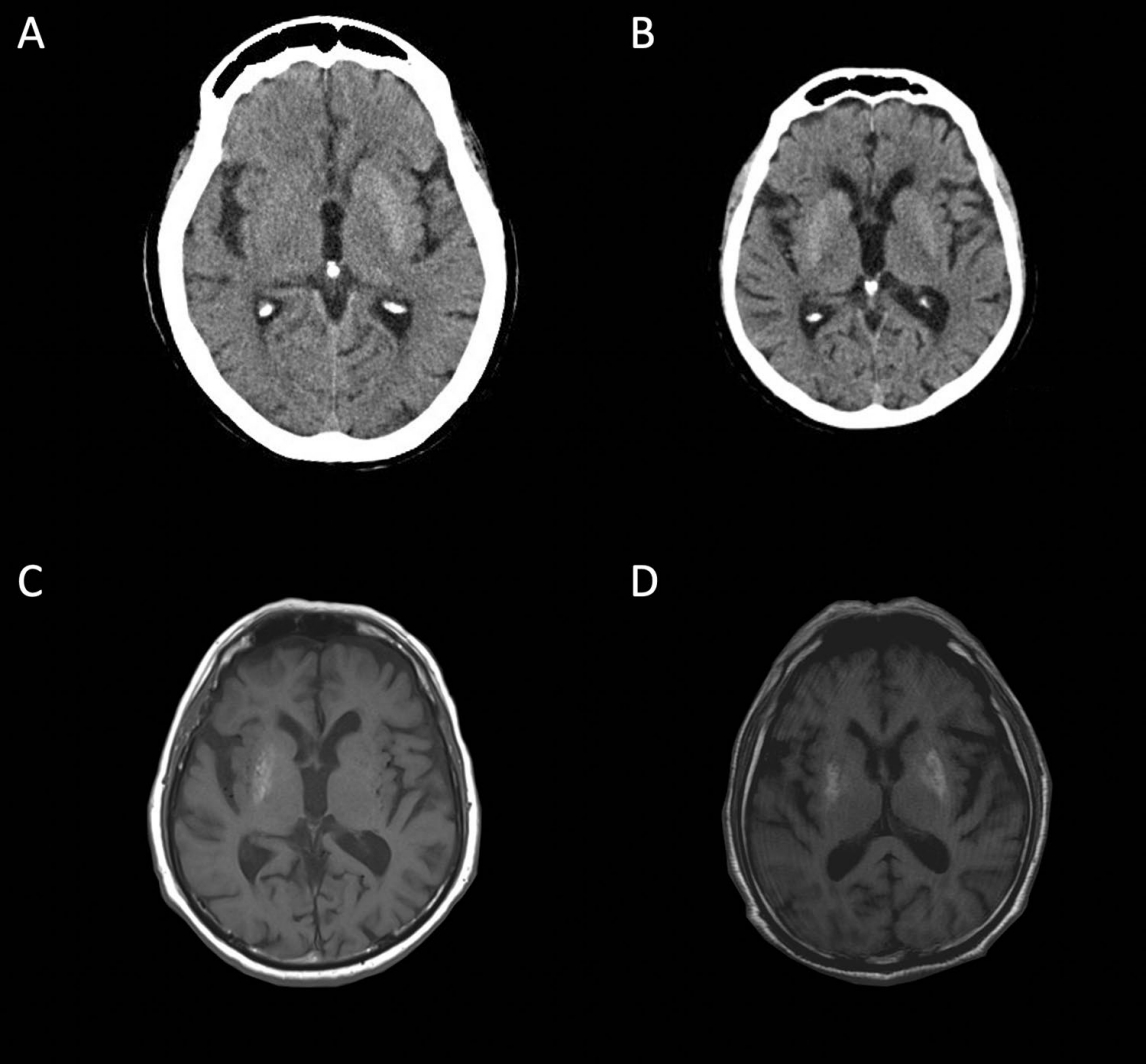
Typical neuroimaging of diabetic striatopathy. A, B Axial CT scan: contralateral caudate nucleus and putamen (A) and bilateral striatal hyperdensity (B). C, D Axial T1-weighted MR image: contralateral (C) and bilateral pallidal-putaminal (D) hyperintensity can be observed
